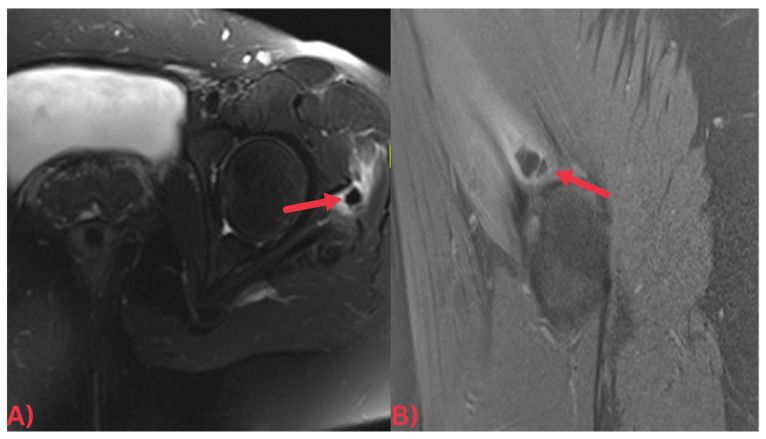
(A) Axial T2 fat-saturated MRI image and (B) sagittal PD fat-saturated MRI image of the left hip demonstrating a well-defined low signal intensity calcification at the insertion of the left gluteus medius tendon (red arrows) with surrounding soft tissue edema.
